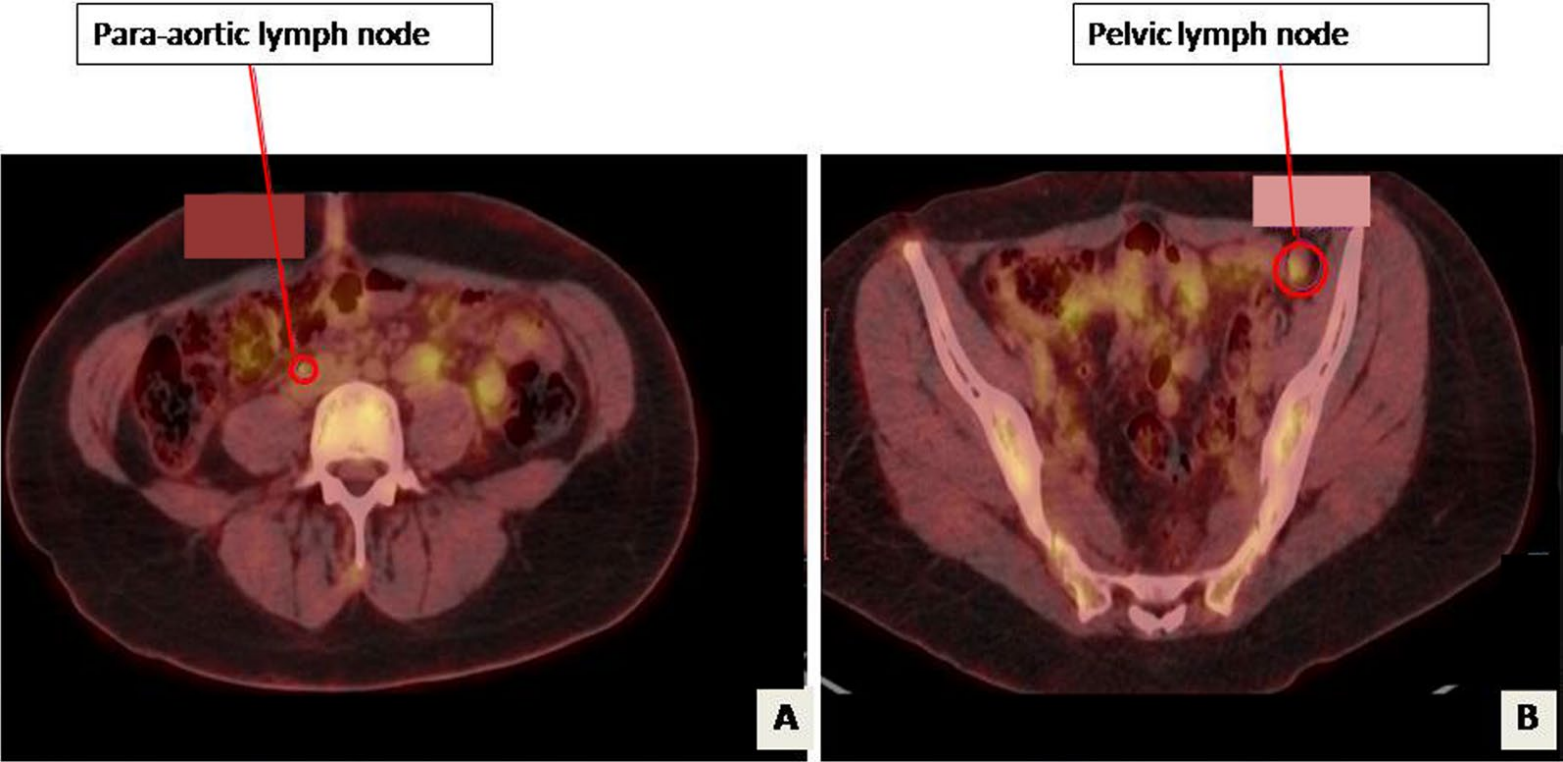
Positron emission tomography-computed tomography findings; A images show intense fluorodeoxyglucose uptake in paraaortic region (appointed with red arrow) and B in the pelvis lying (appointed with red arrow) along external iliac arteries
